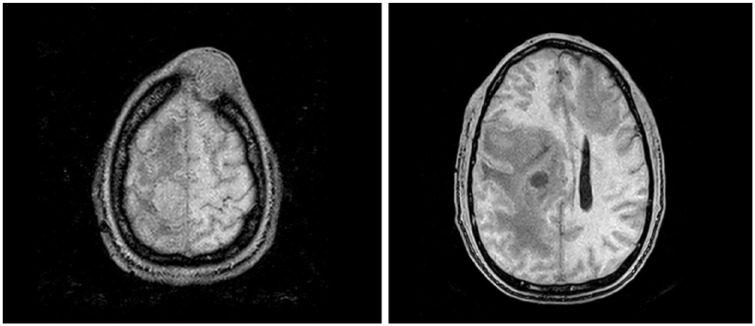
Magnetic resonance imaging showing the primary scalp lesion and the metastatic brain lesions.
